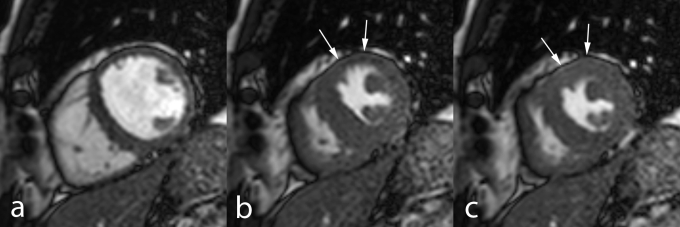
MRI study (dobutamine–atropine stress) in 60–year–old man. Midventricular cardiac short–axis cine MRI at end–diastole (a), end–systole (b), and isovolumic relaxation (c). At maximal stress, during systole (b), the ischemic myocardium in the anterior wall is akinetic (*arrows*). However, during isovolumic relaxation (c) while the non–ischemic regions start to relax, myocardial thickening can be is seen in the anterior LV wall (*arrows*), i.e. post–systolic contraction of the ischemic myocardium. Coronary angiography showed significant stenosis in the proximal LAD coronary artery.
